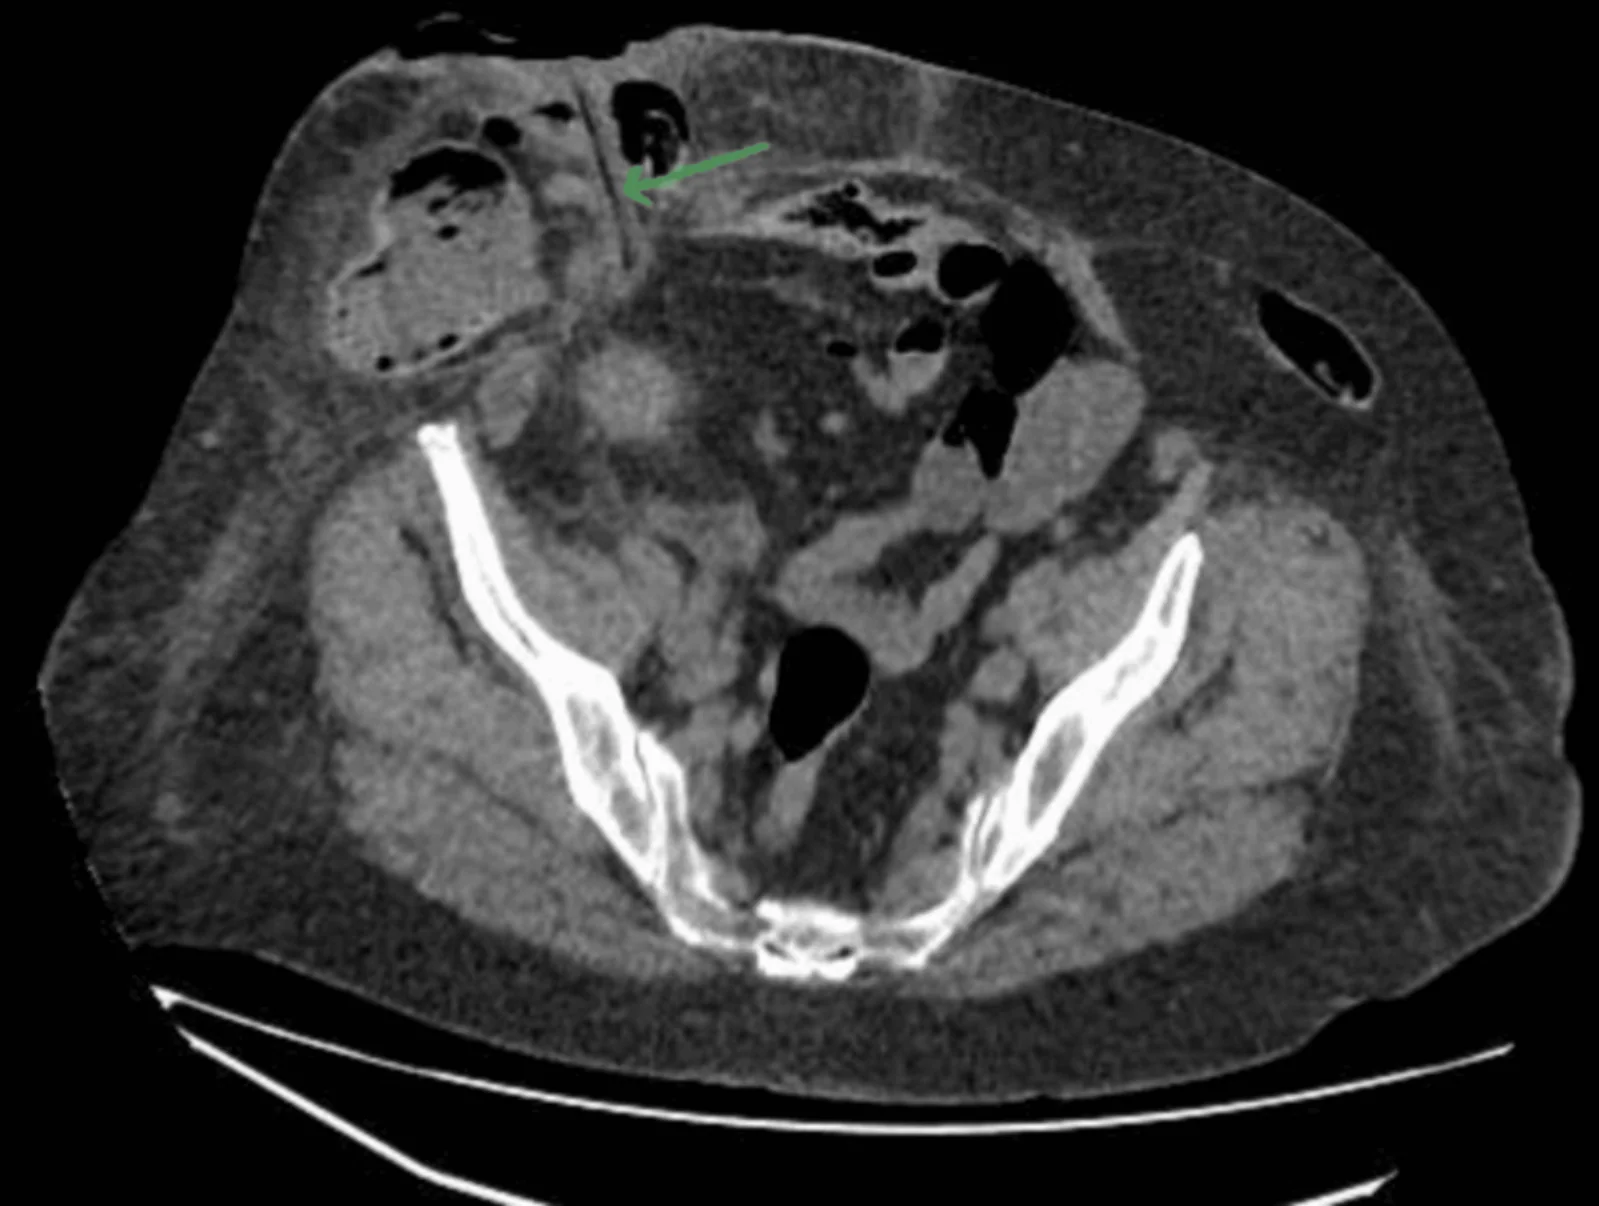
Axial view of CT abdomen The image shows a catheter (green arrow) within the ileal conduit in the anterior abdominal wall CT: computed tomography
